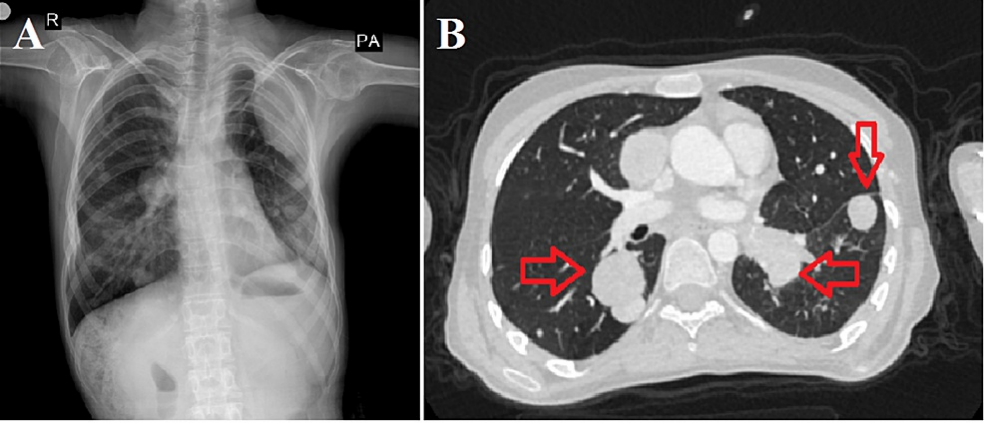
Posteroanterior chest X-ray and thorax computed tomography images of pulmonary metastatic nodules and foci A: Posteroanterior chest X-ray. B: Transverse section view of thorax computed tomography images of pulmonary metastatic nodules. Red arrow: pulmonary metastatic nodules.
